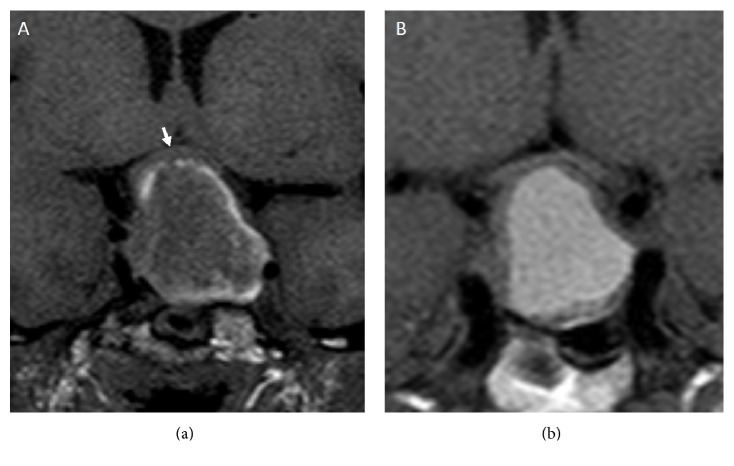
Coronal T1-weighted MRI images in two patients with pituitary apoplexy showing (a) intrasellar and suprasellar mass with a peripheral high signal intensity ring due to methemoglobin and slightly compressing the optic chiasm (white arrow) and (b) intrasellar and suprasellar mass with diffuse central high signal intensity.
